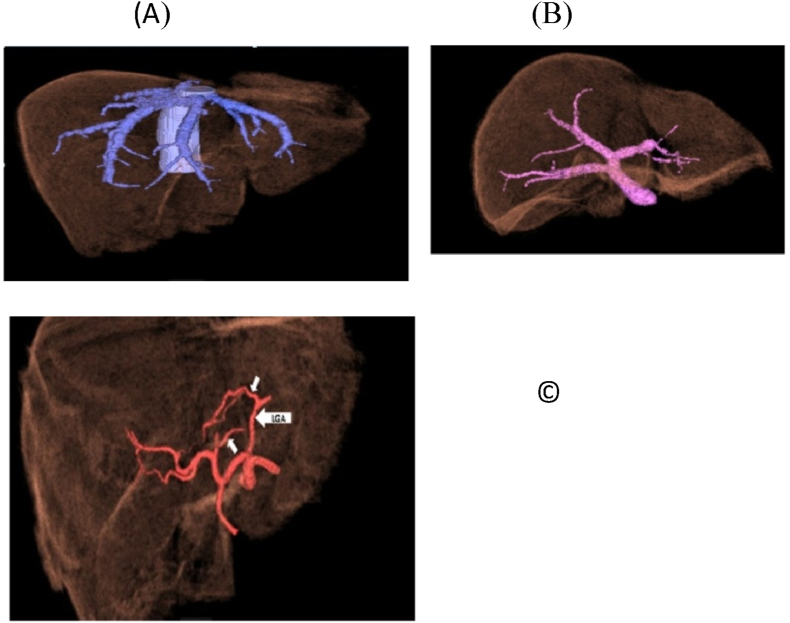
CT with hepatic protocol and 3d imaging reconstruction showing: A: separate left HV. B: single left PV. C: 2 left HAs.
